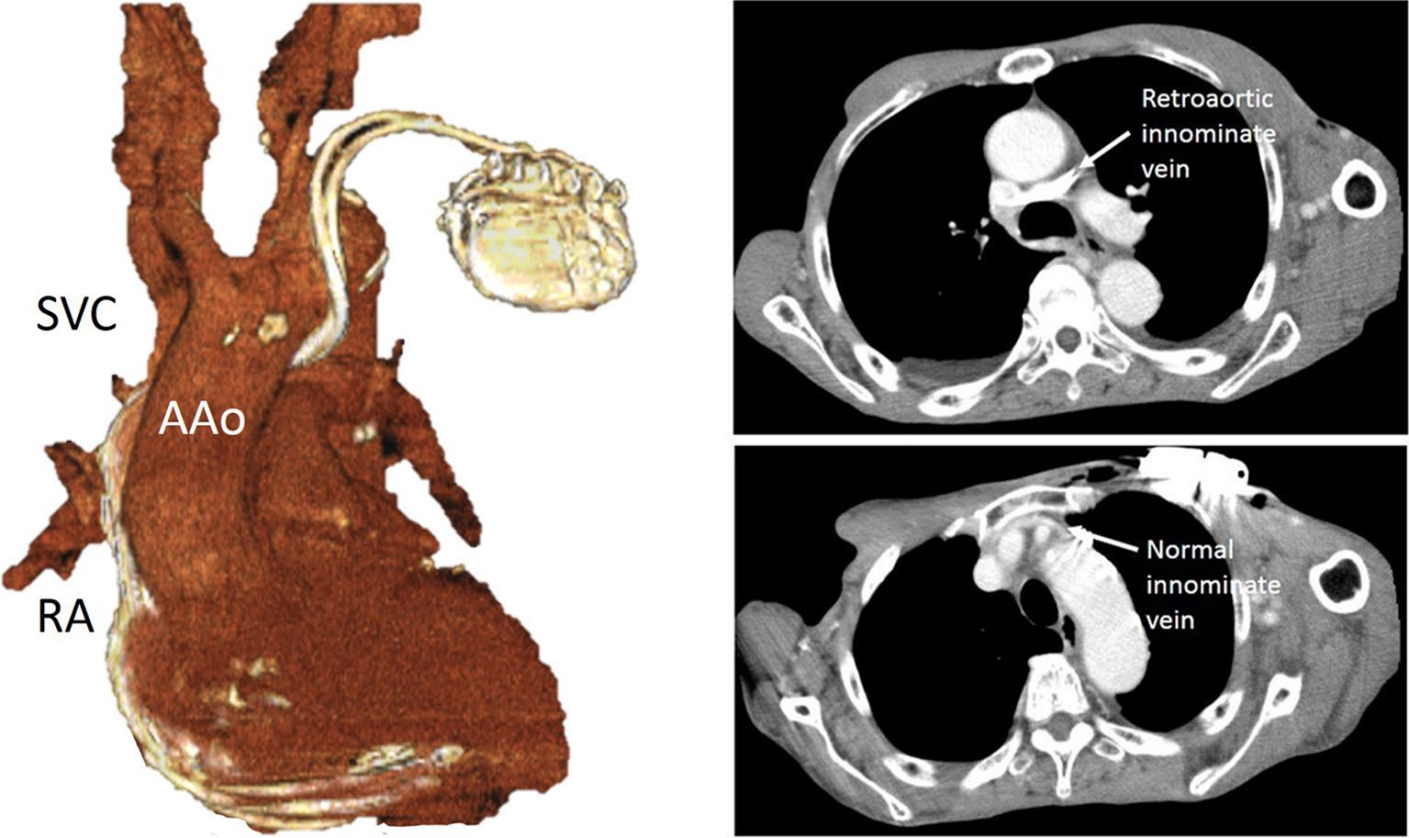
Computed tomography revealed duplication of left innominate vein. There was no other abnormality
